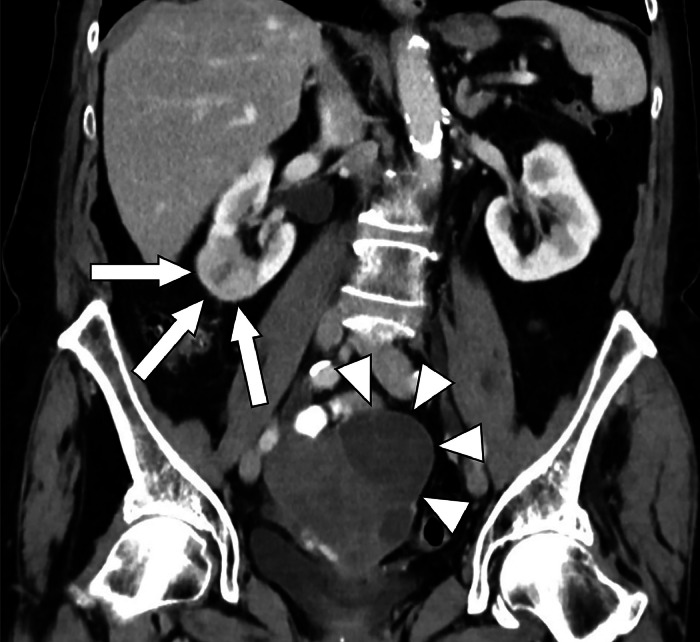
Initial imaging on February 10, 2023, by an external radiologist. Contrast-enhanced abdominal CT in venous phase, coronal reformation. This CT is showing a suspicious mass at the lower pole of the right kidney with central hypodensity (white arrows) and an inhomogeneously enlarged uterus with cystoid changes (white arrowheads).
